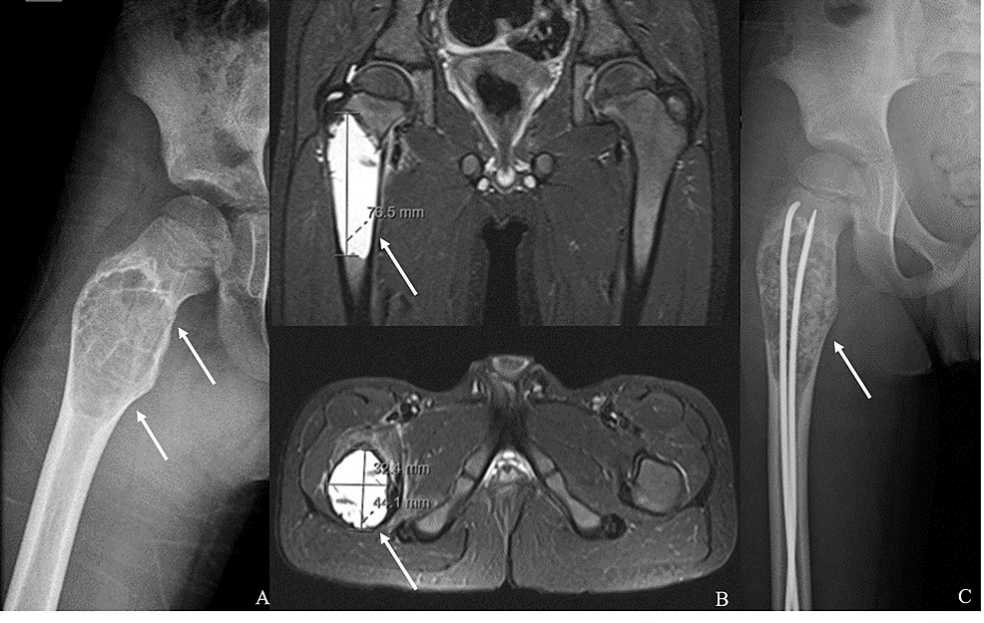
A) Radiograph: cystic lesion of the proximal right femur; B) MRI: expansive lesion, on longitudinal and transversal planes; C) Radiograph after surgery: intramedullary nails image
